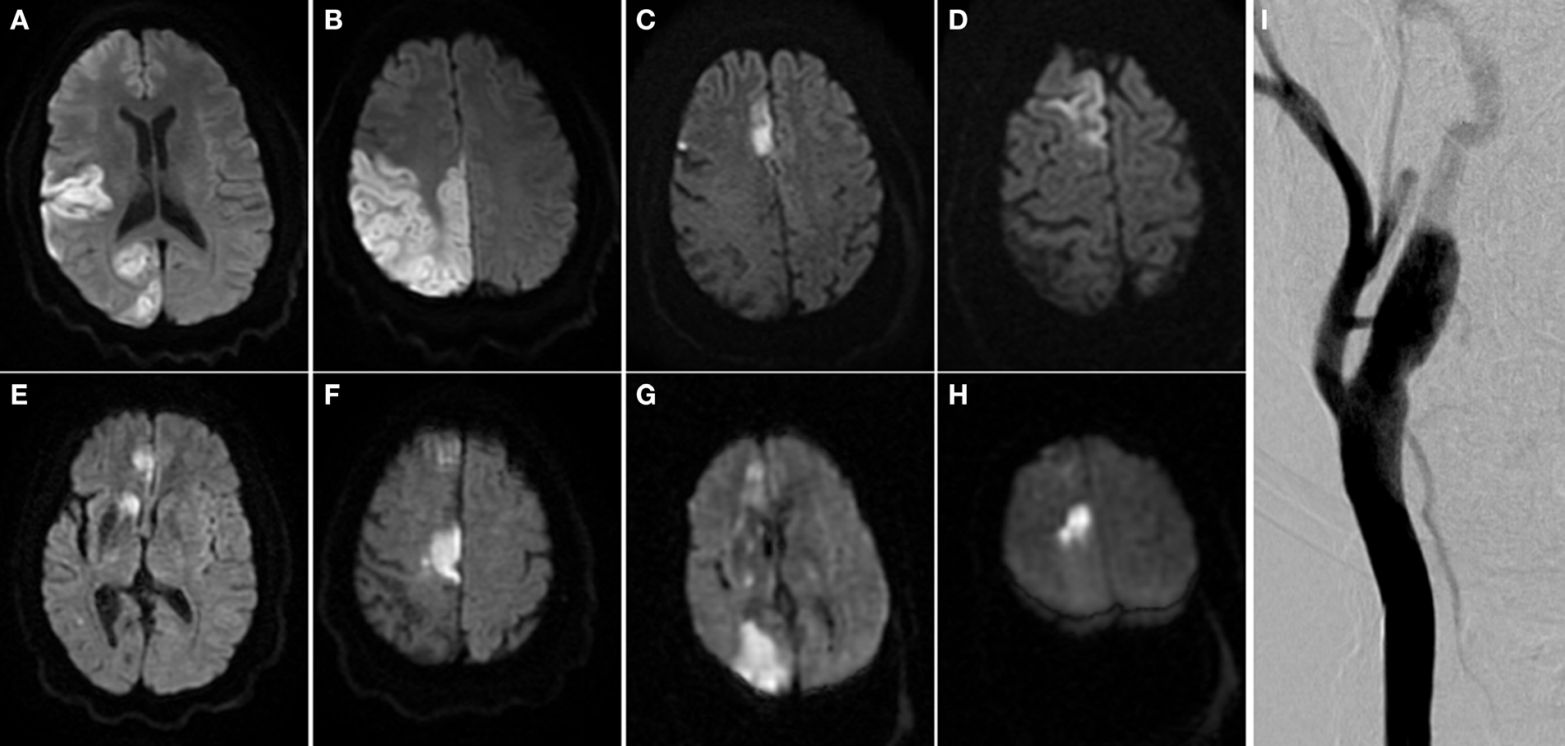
Multiple embolic territory strokes secondary to irregular and vulnerable carotid atherosclerotic plaque. (A–H) Brain MRI scans from multiple ischemic events over multiple admissions. (I) Right carotid angiogram. Arrow indicates atherosclerotic plaque in right internal carotid artery.
